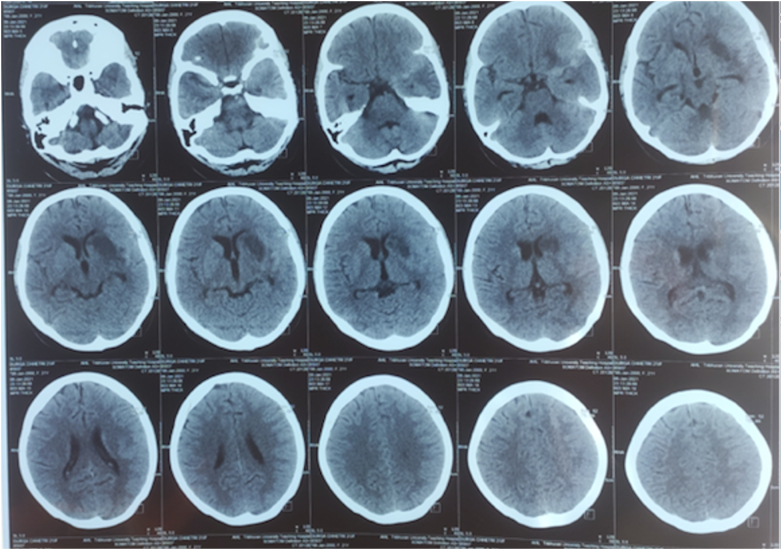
Plain CT head which shows acute subarachnoid hemorrhage involving left Sylvian fissure with hypodensity around left basal ganglia suggestive of vasospasm.
